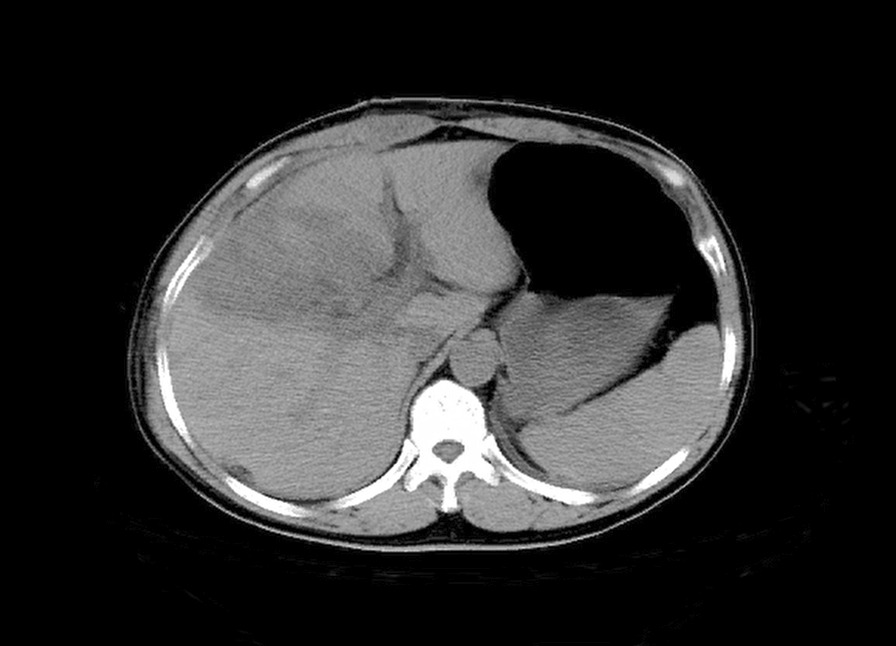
Postoperative abdominal CT scan
